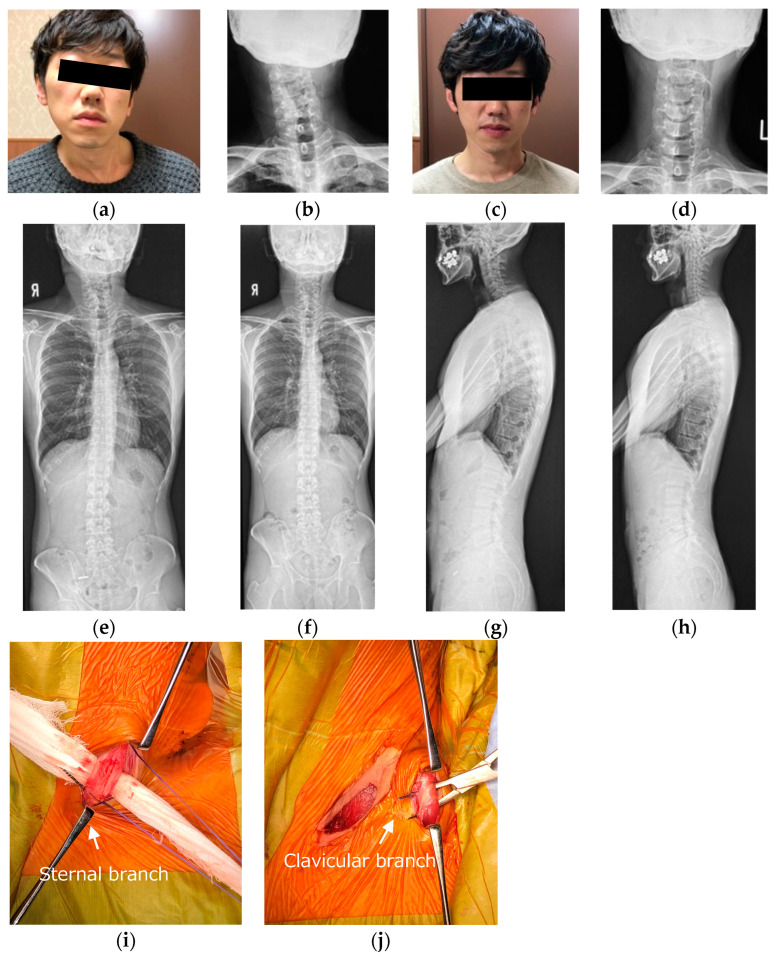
Forty-year-old male was treated with unipolar release of the sternocleidomastoid. Pre- (a,b,e,g) and postoperative (c,d,f,h) appearance, radiographs, and intraoperative photographs. The head was markedly tilted to the left side, and the neck was shifted to the right side preoperatively. Left lower ends of the sternal branch and clavicular branch of the SCM were released at two incisions (i,j). There were good improvements in appearance and postoperative X-rays after the unipolar release of SCM.
